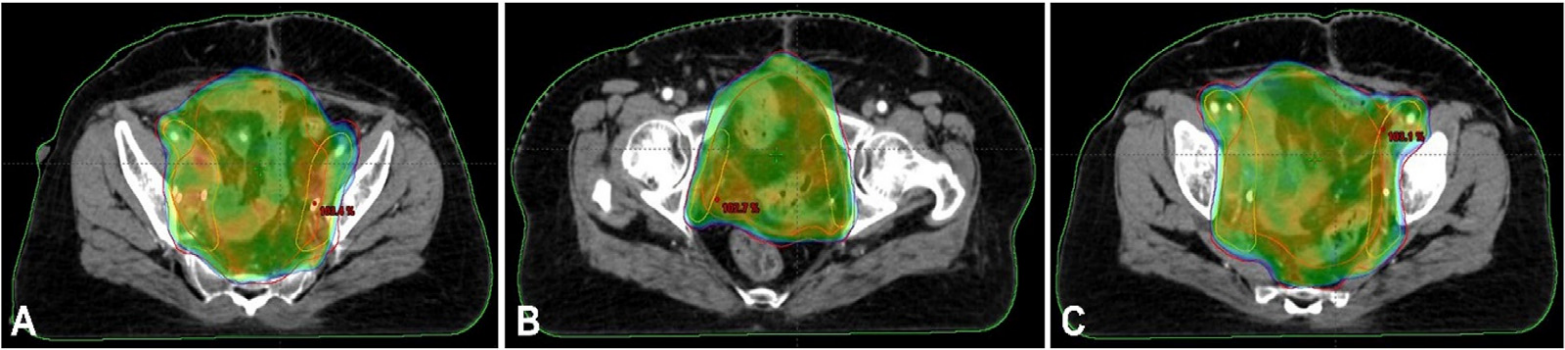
PET-CT based radiation therapy planning for bladder carcinoma.
